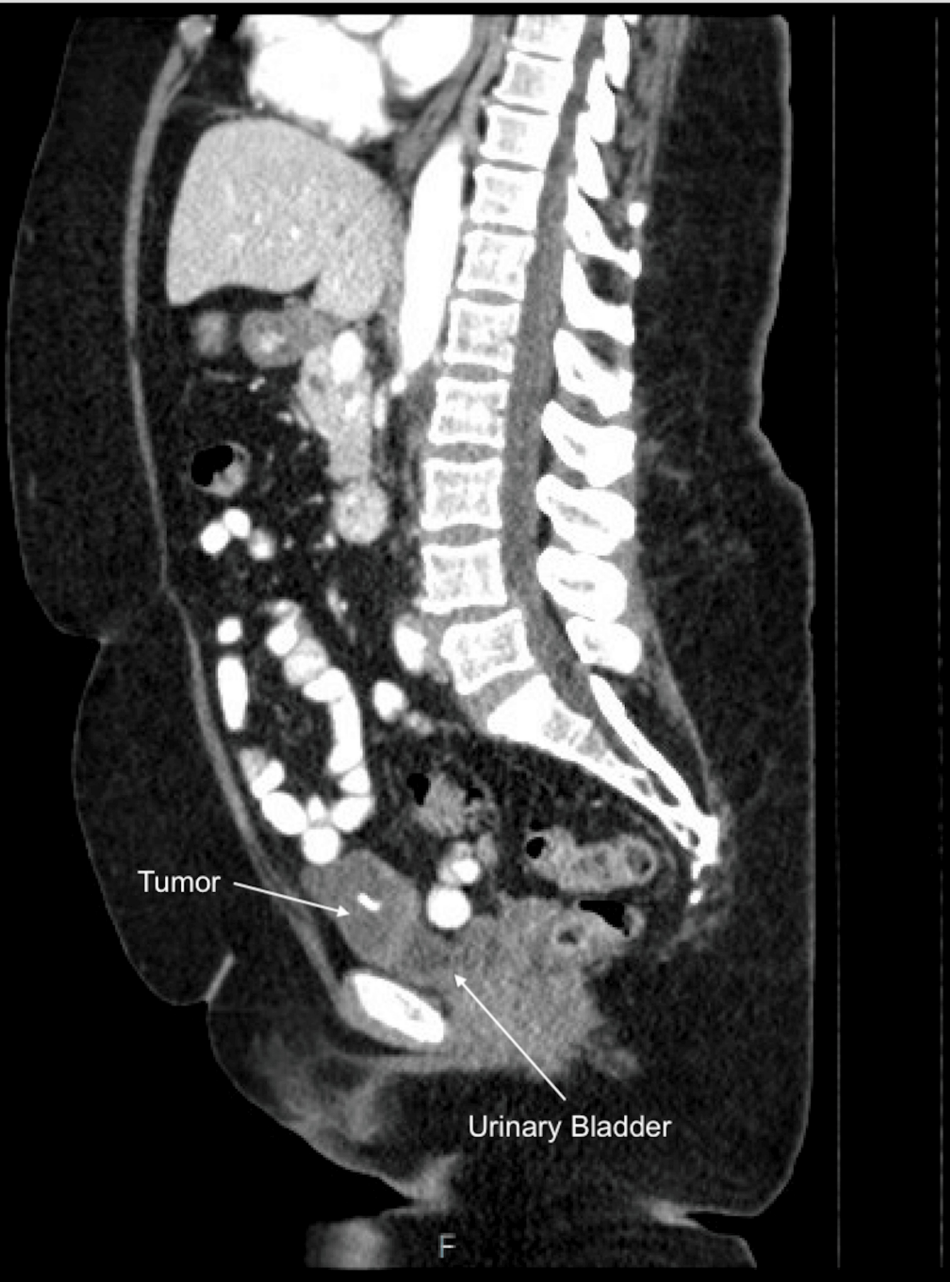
Sagittal CT of the abdomen and pelvis re-demonstrating the urachal mucinous cystadenocarcinoma in relation to the urinary bladder. CT: computed tomography
